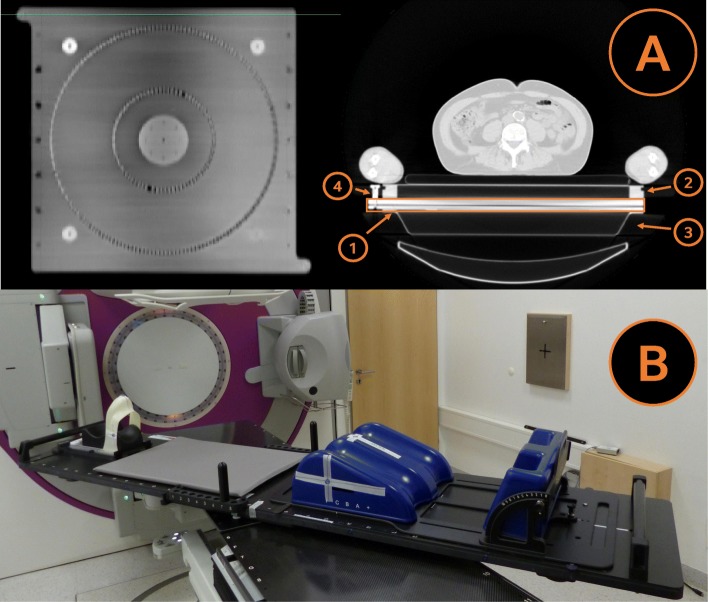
Coronal (a left) and axial (a right) CT-scan of the ball bearing rotational unit (1), holding the TBI-tabletop (2) on the linac couchtop (3). Carbon fixation buttons (4) lock the unit in head-first and feet-first positions to prevent unintended rotation. b shows the tabletop on the linac couchtop with integrated immobilization components
